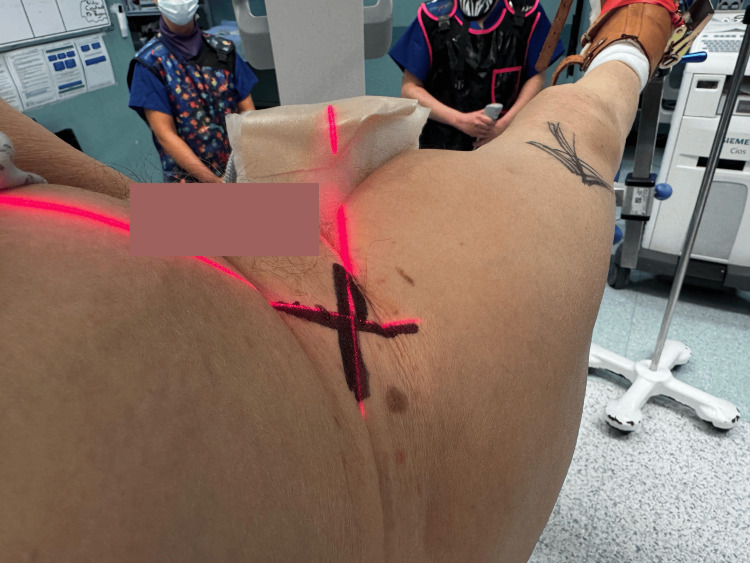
Laser position marked with a permanent marker The laser position is marked with a permanent marker for reference and to guide the radiographer to reposition the image intensifier for subsequent images.
